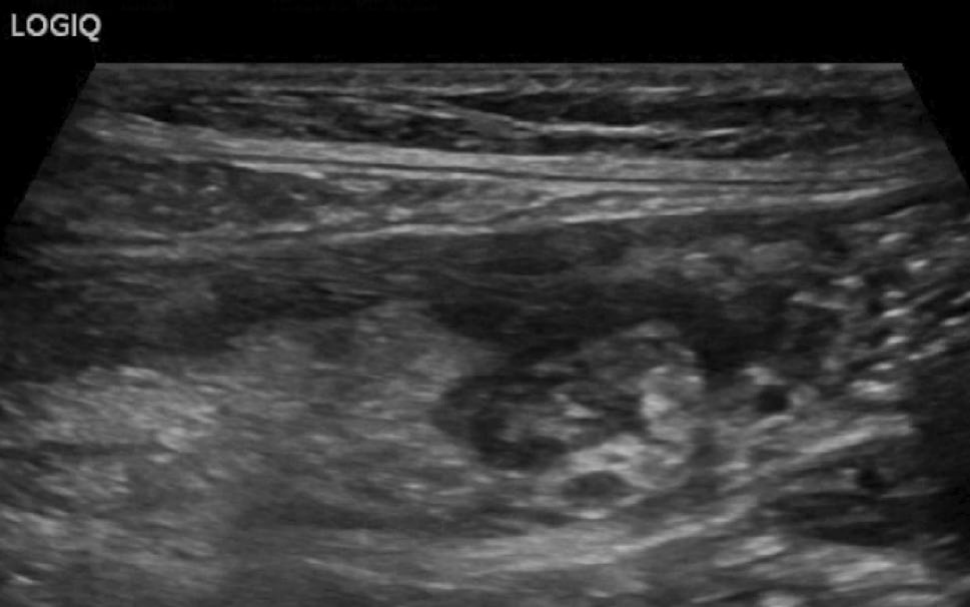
At the level of the right colon, just before the cecum, there is a hypoechoic outpouching measuring 1 cm, with a thickened wall (5 mm) and abundant vascularization on color Doppler imaging
